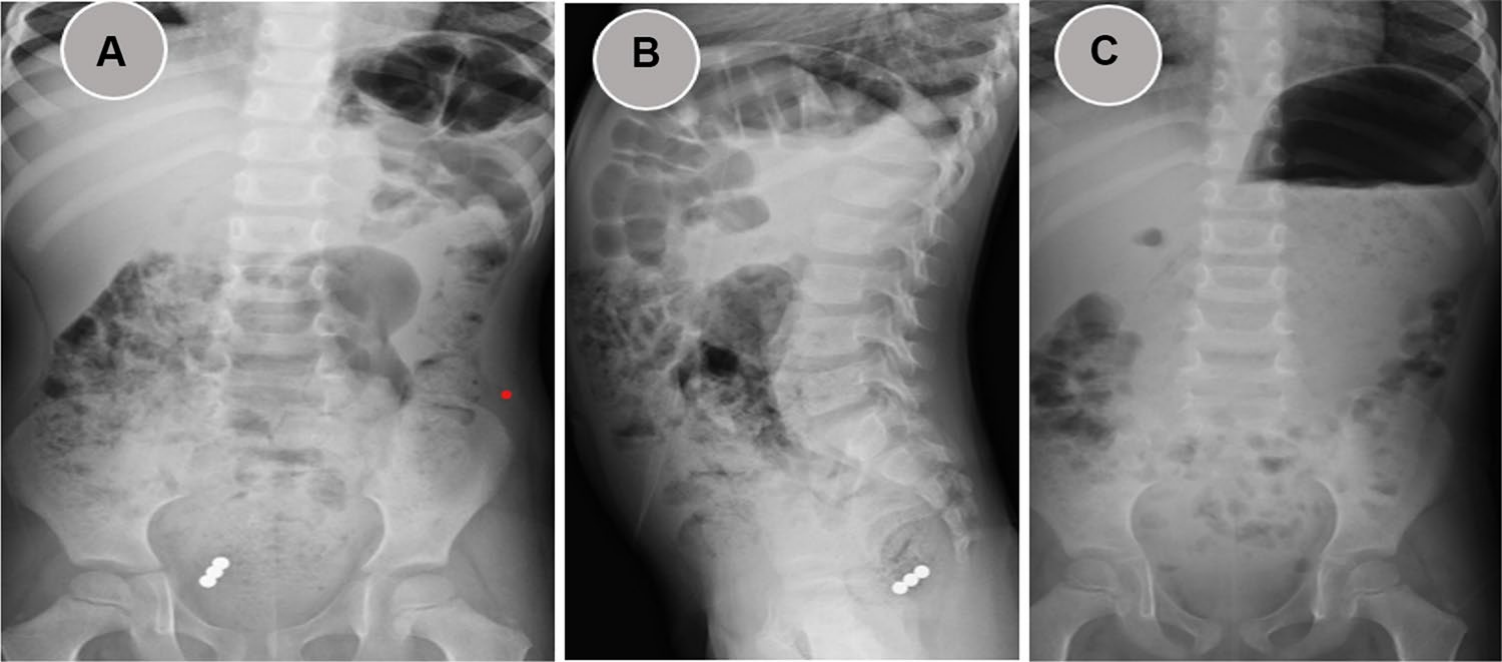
Radiograph showing 3 attached magnets without intervening tissue passed naturally (A) AP view; (B) Lateral view; (C) after evacuation of magnets.
